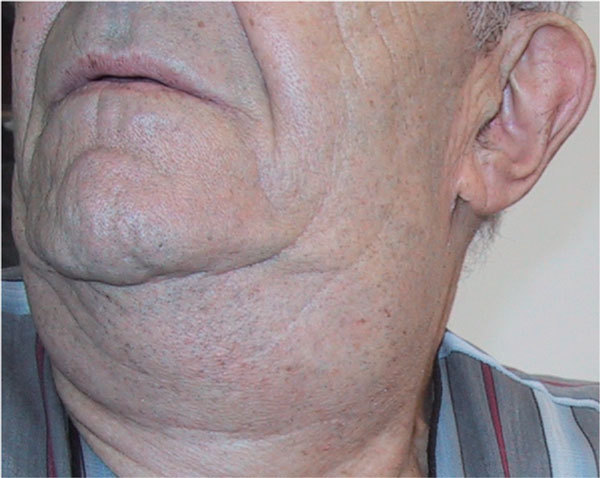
Swollen parotid gland 24 hours after a lung CT scan with an NICM.
